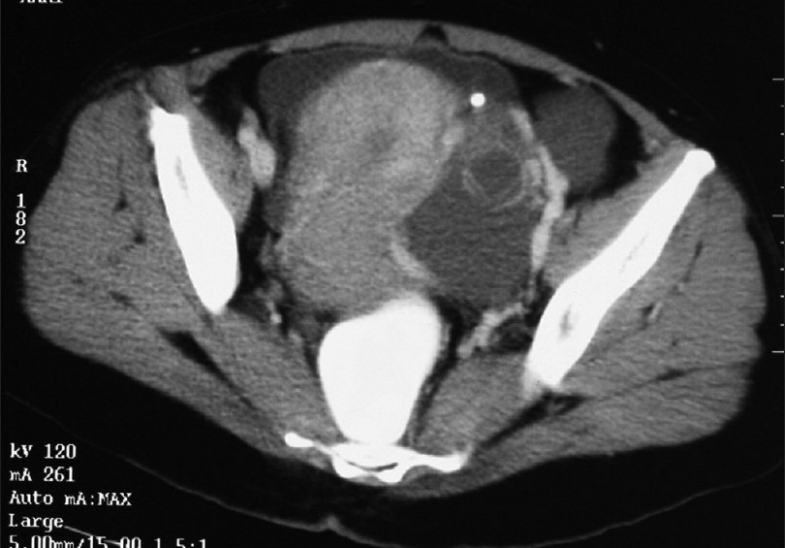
Contrast-enhanced CT scan showing tumor with increased vascularity in the periphery
